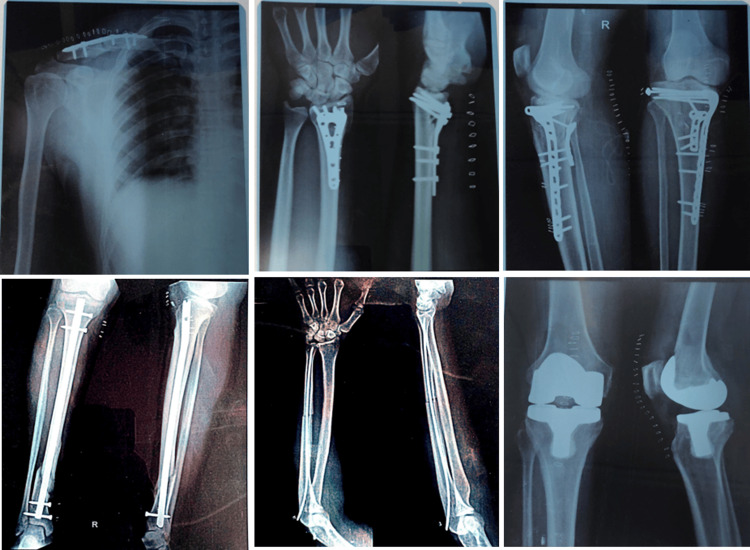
Post-surgical X-ray examination shows no traces or residue of Surgi-ORC® on day two
